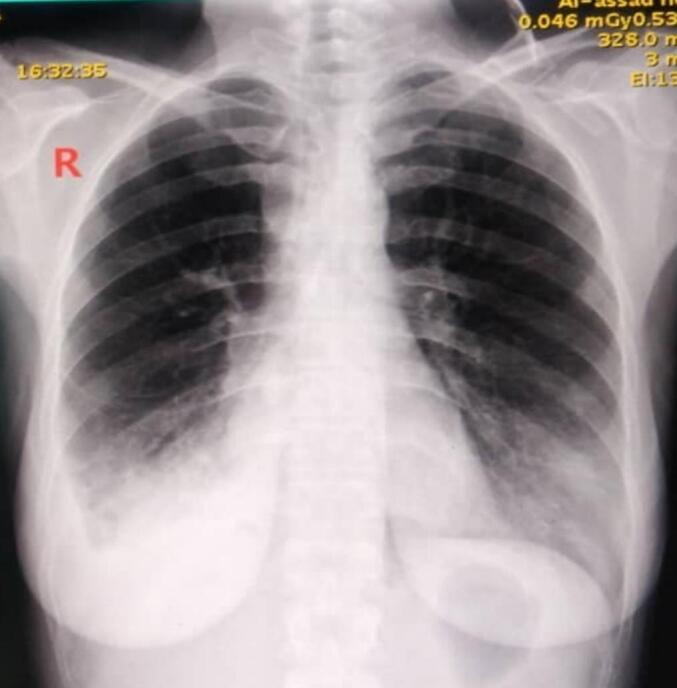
Chest x-ray showing A shadow in the trachea with bilateral pulmonary infiltrates and right angle closure
